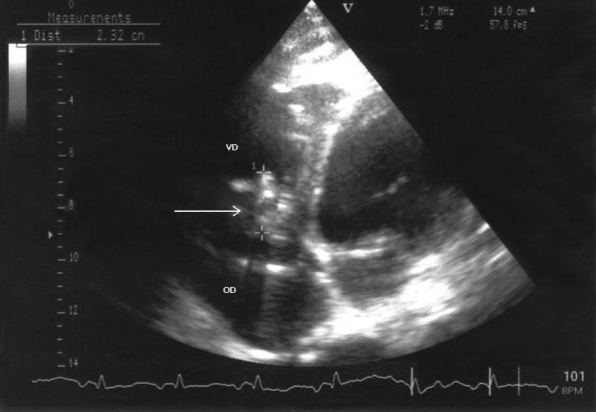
Transthoracic echocardiography at time of diagnosis showing large vegetations on the tricuspid valve caused by *Tropheryma whipplei*. The tricuspid valve is indicated by an arrow. VD; right ventricle: OD; right atrium.
